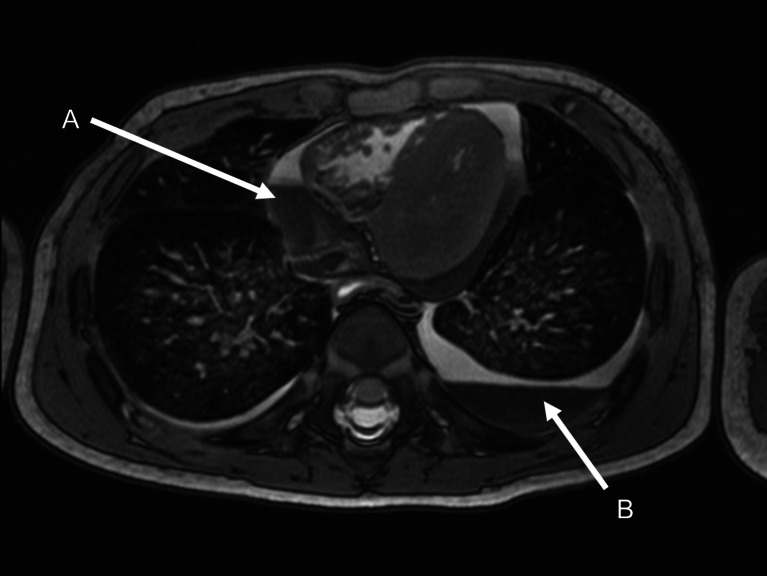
Postmortem axial MRI of the chest of an 8-month-old boy who died of unknown causes. Unusually, there was apparent sedimentation/layering of blood in the pericardial sac (a) as well as in the left pleural cavity (b), which was attributed to traumatic pericardiocentesis during resuscitation. There was no evidence of trauma
